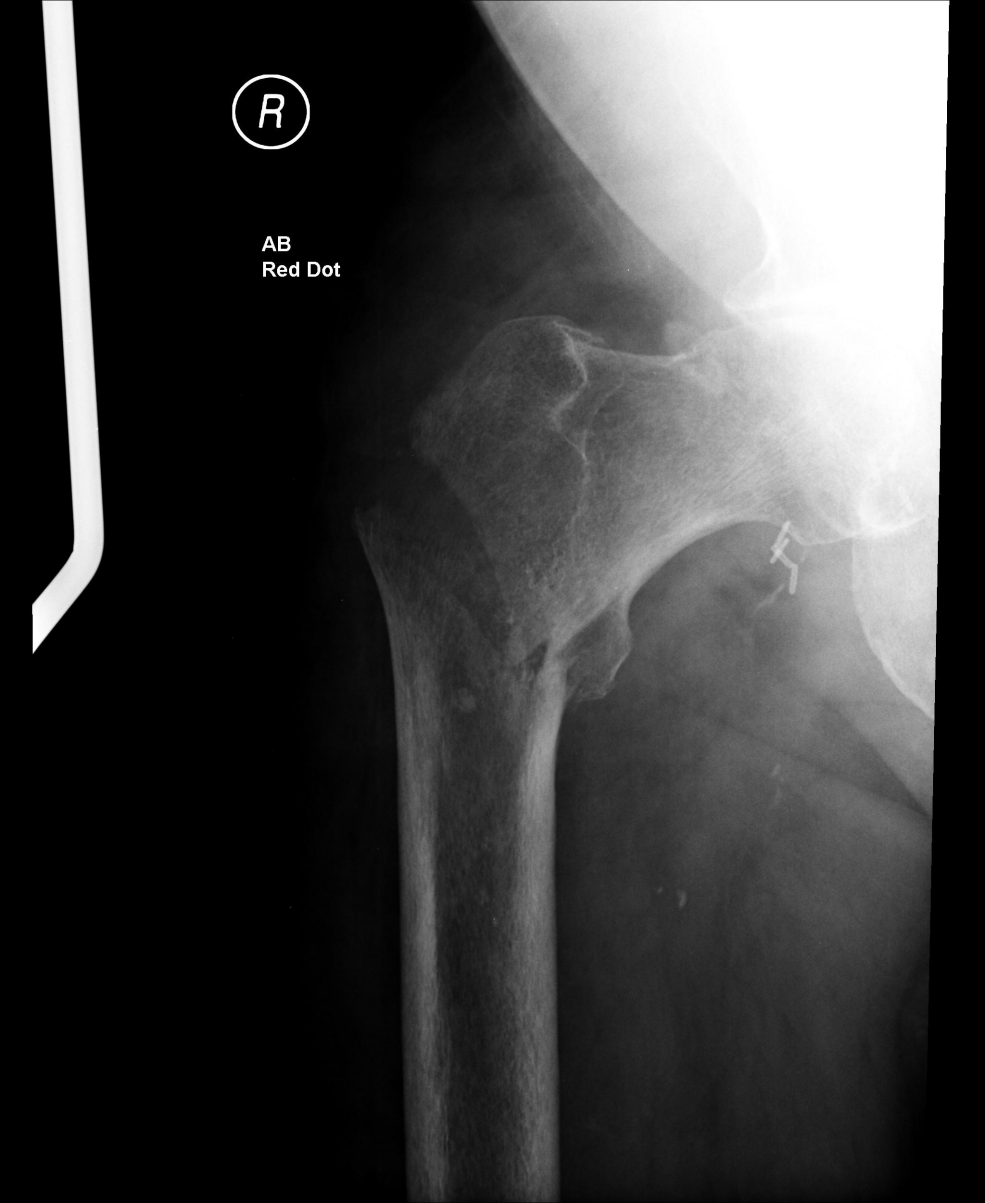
Preoperative plain radiograph of the pelvis showing the intertrochanteric fracture of the right femur.
